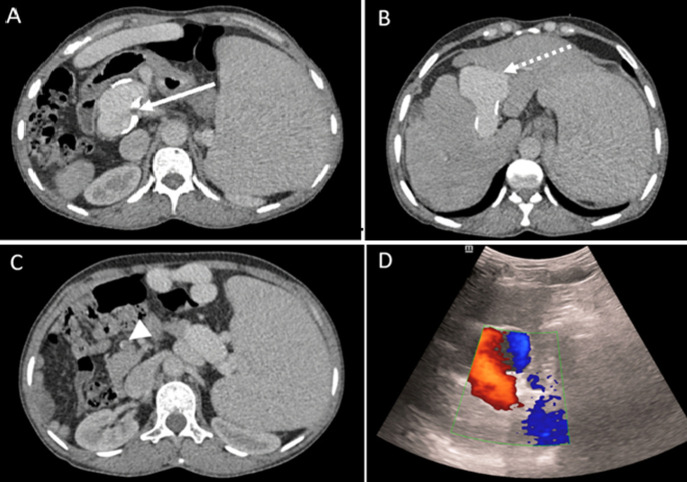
portal venous system abnormalities and thrombosis; A-C) axial CT images showing a dilated portal vein with parietal calcifications; A) a fusiform aneurysm in its intrahepatic segment; B) thrombosis of the superior mesenteric vein; C) Doppler ultrasound; D) a hepatofugal and bidirectional flow pattern
